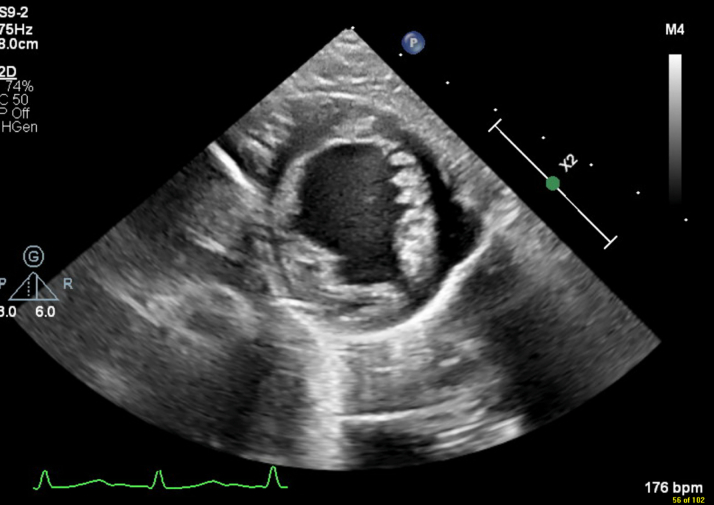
Initial Echocardiogram Echocardiogram (parasternal short axis view) from initial visit.
